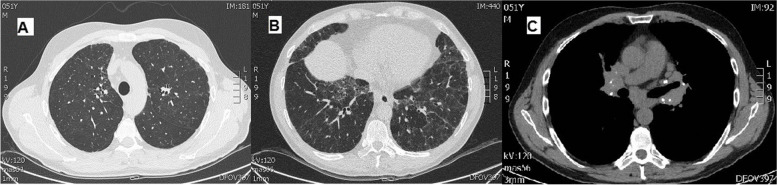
Chest HRCT showing ill-defined centrilobular opacities in the upper lobes (A); normal density areas, extensive ground glass attenuation and lobular areas of air-trapping (“head cheese pattern”) with reticular zones of fibrosis in the lower lobes (B). Mediastinal window shows hilar and mediastinal lymph nodes enlargement with small calcifications (C)
